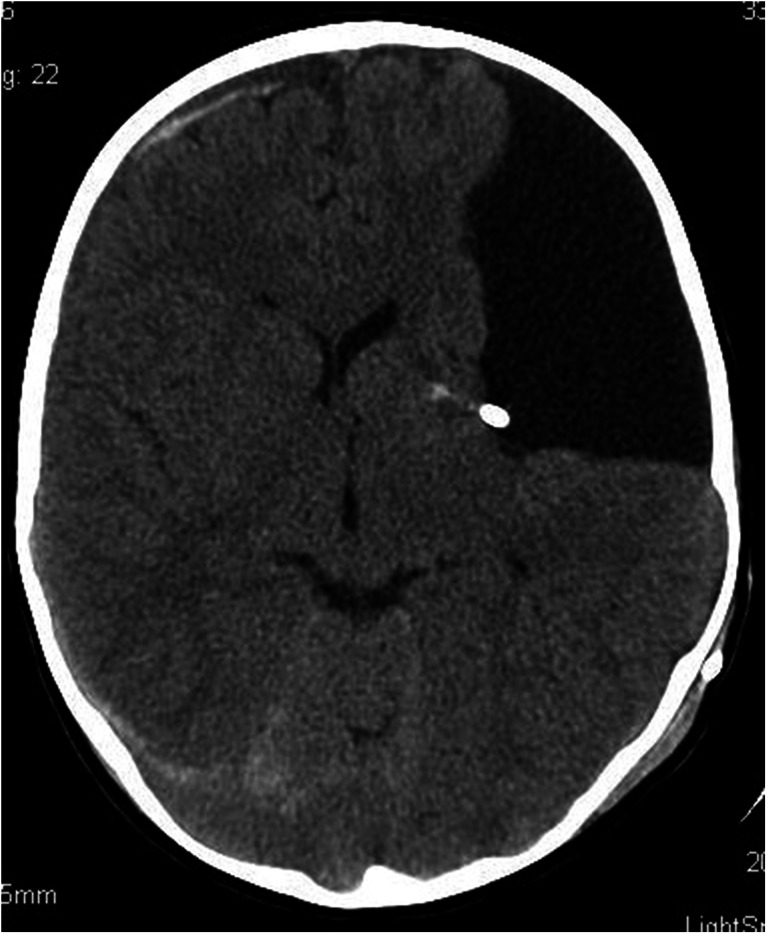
The photo presents the same scan as in Fig. 3, but a different cross section. A small subdural hygro-hematoma in the right frontal region. A blood trace along the tentorium of the cerebellum. A mass effect seen as minimal displacement of the lateral fissure of the brain to the right, with mild compression of the frontal corn of the left lateral ventricle. Nonenhanced CT scan
